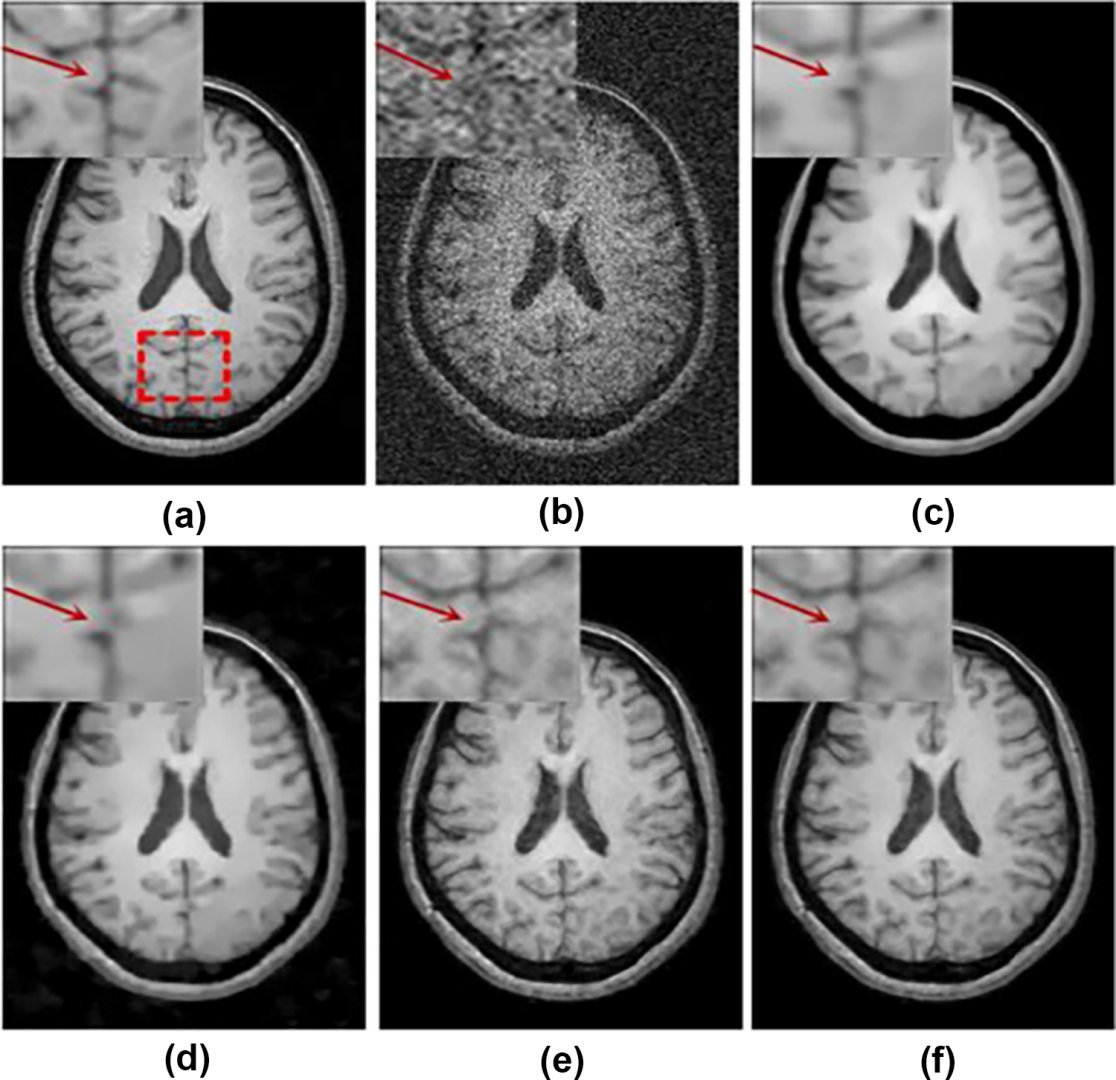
Comparison of image denoising on a T1-weighted image [30]: (a) noise-free image, (b) noisy image, (c) BM4D, (d) PRI-NLM3D, (e) CNN3D, and (f) RED-WGAN [48]
